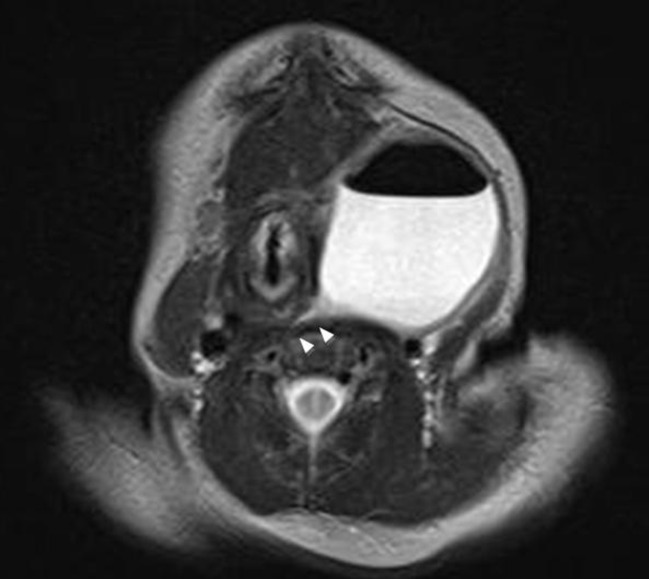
Figure 2: Neck MRI Findings(T2): A 4 × 4 × 3.7 cm size well-defined unilocular cystic mass in the left infrahyoid neck. An internal air-fluid level with suspected fistulous tract at the level of the left pyriform sinus and an inferomedial beak at the level of the cervical esophagus is shown. (Arrows)
